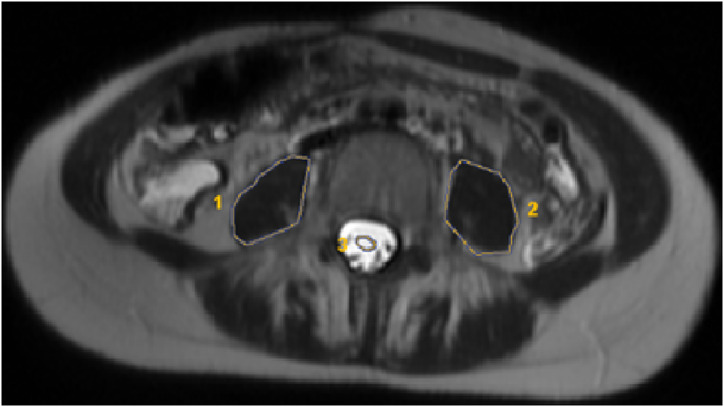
The CSF and psoas intensity were measured with the free-hand ROI method at the same level on the axial T2-weighted images. CSF, cerebrospinal fluid; ROI, region of interest.
